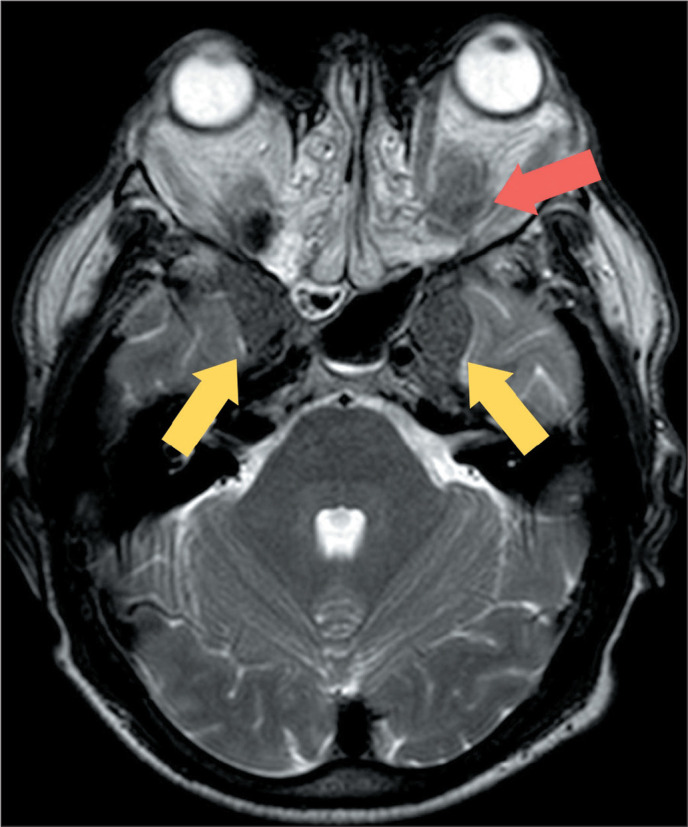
MRI of a patient with IgG4-related disease. T2WI of the skull, showing
